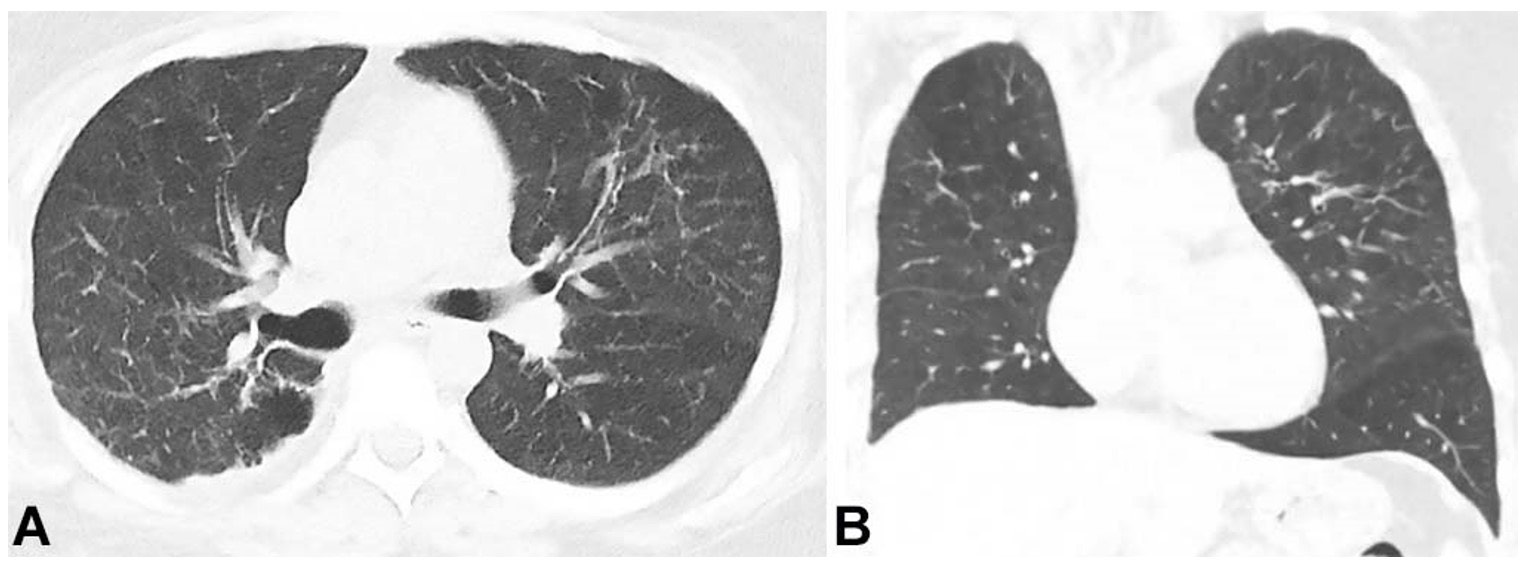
Chest CT. A – axial plane, B – Coronal plane; showing a marked decrease of the patchy ground-glass attenuation and interstitial thickening in both lungs​.
